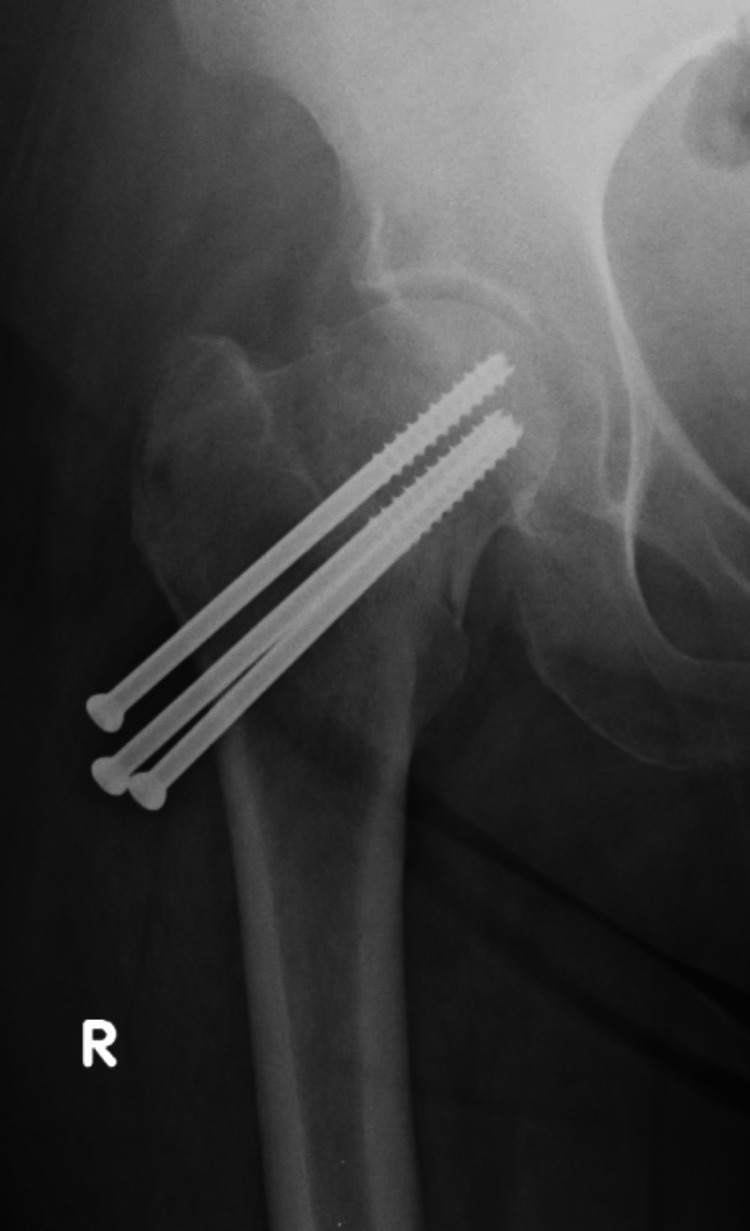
AP X-ray shows non-union with fracture collapse AP: anteroposterior
